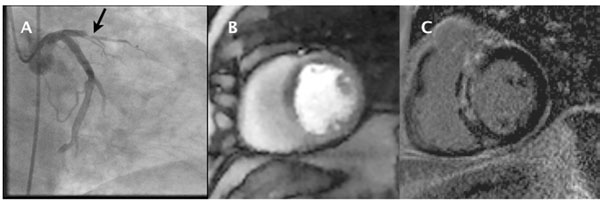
X-ray angiogram of STEMI (A) with 100% occlusion of the LAD and without any other significant flow-limiting coronary lesions. Post-PCI dynamic self-gating perfusion imaging showed a large anterior wall defect (B, rest not shown). LGE confirms a large anterior wall infarct resulting from the STEMI (C).
